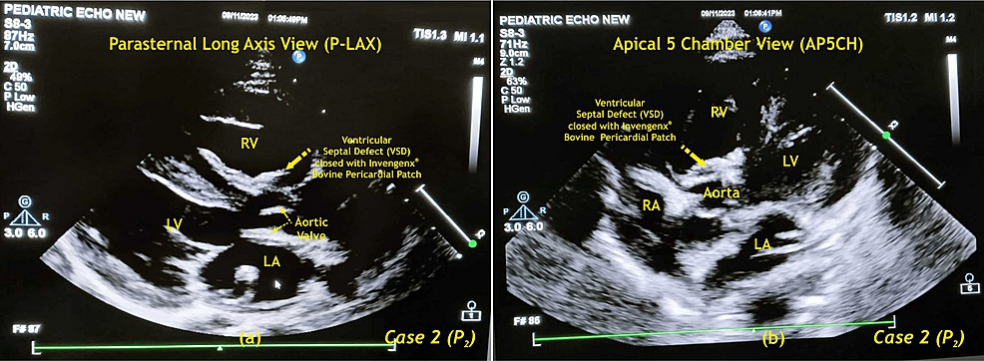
Postoperative 2D echocardiography of case two (P2) RA - right atrium; LA - left atrium; RV - right ventricle; LV - left ventricle; P - patient Image credits: Dr. Vishal V. Bhende
